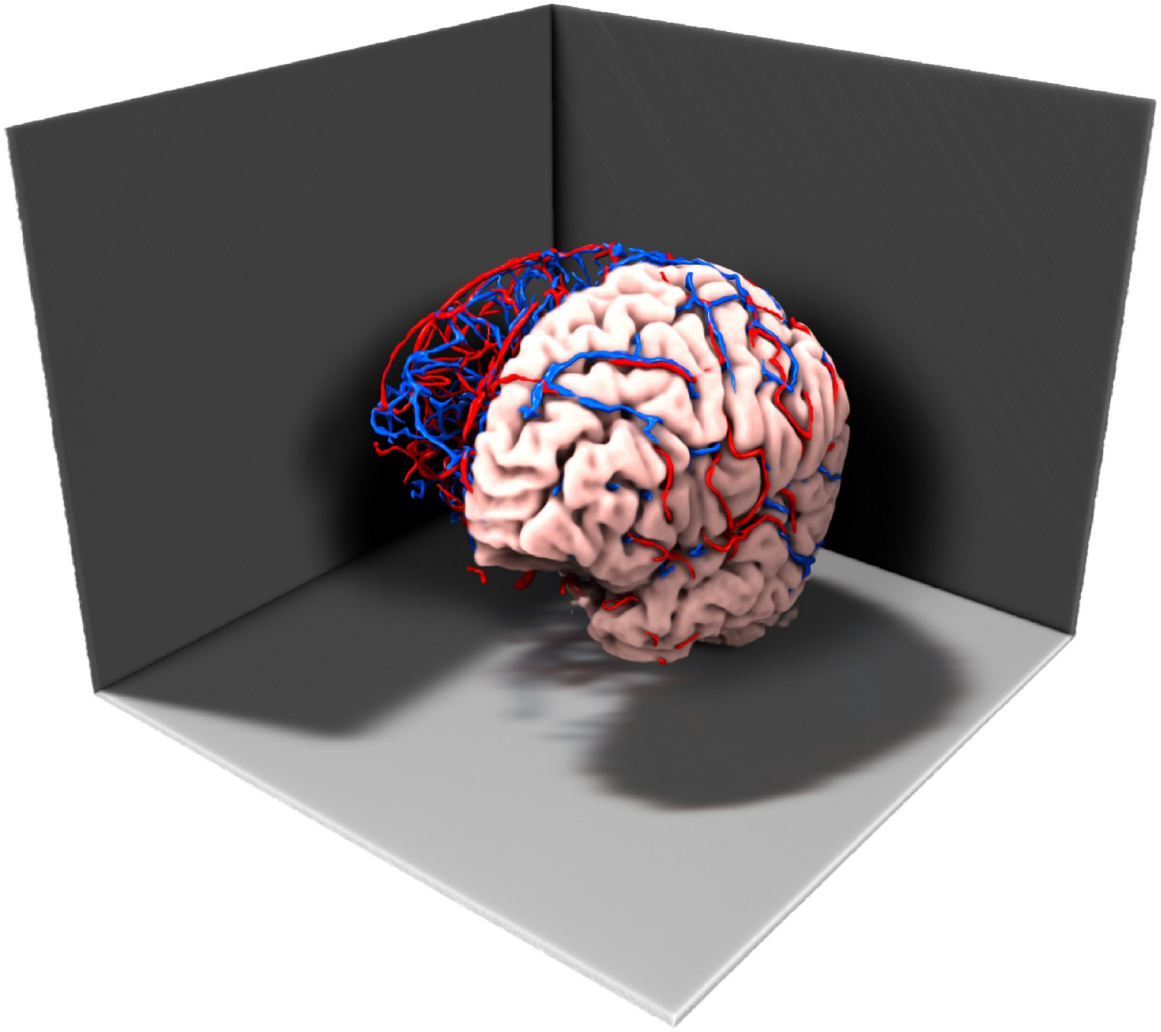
3D volume rendering of the T1-weighted data including the arterial (red) and venous (blue) vessels.
